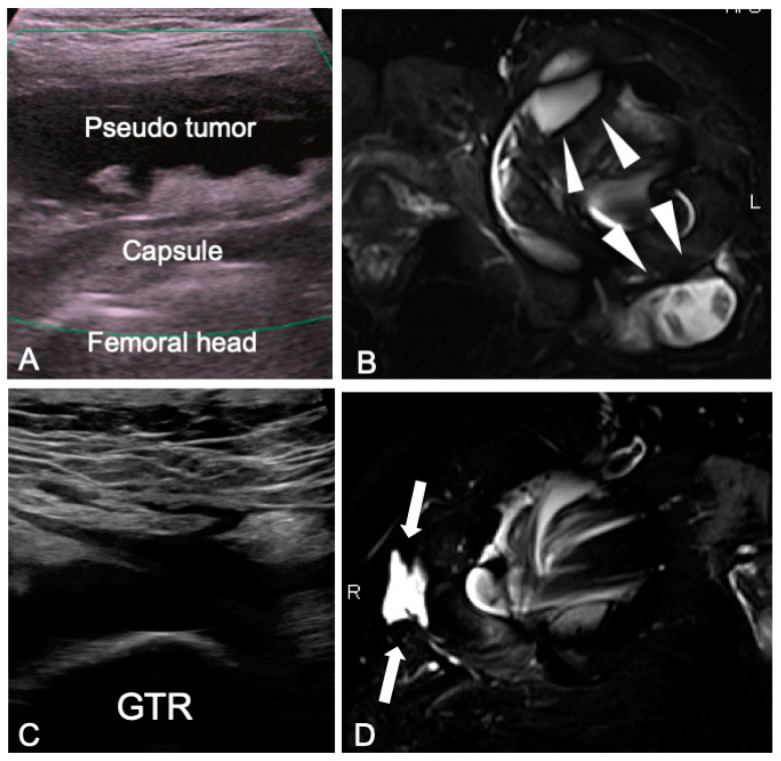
Representative abnormal images taken using ultrasonography and MRI in the hip resurfacing patients. (A,B): A pseudotumor was detectable in the anterior long axis of the hip by ultrasonography as a free echoic space above the femoral head (A). MRI provided information regarding the area of spread and lesion properties (white arrow heads) that is vital for the preoperative assessment of the pseudotumor (B). The trochanteric synovium was detected by ultrasonography in a patient with lateral thigh pain as a free echoic space located above the greater trochanter (C). MRI also provided information on local fluid collection (white arrows) at the lateral side of the greater trochanter (D).
